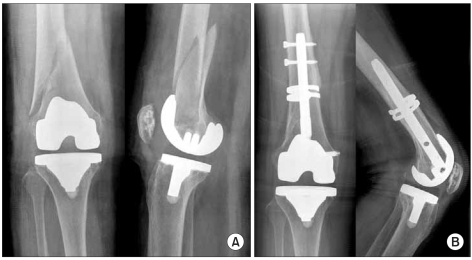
(A) Preoperative X-rays of a periprosthetic fracture of the right knee in a 57-year-old woman. (B) X-rays taken 5 months later show mature callus formation. A short supracondylar nail was used due to femoral anterior bowing.
